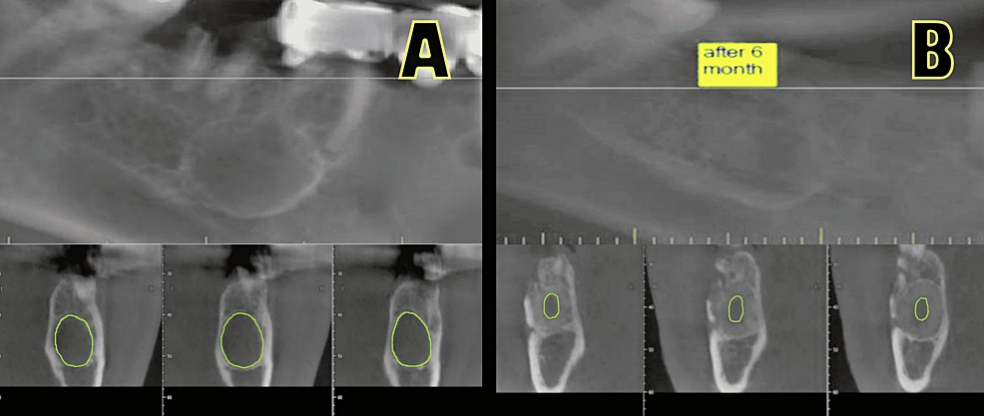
Radiographic assessment. A: A well-defined radiolucent lesion present apically to tooth 46 is visible on the presurgical one-beam computed tomography (CBCT) scan. lesion covered in the buccal cortical plate. B: CBCT scan, six months after the surgery. Good bone defect filling was noted, and the bone lid is integrated.
